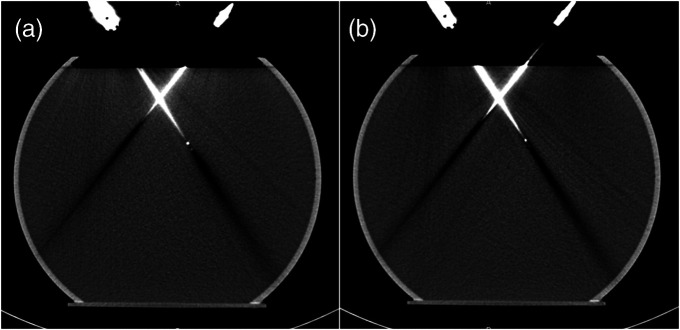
Comparison of probes placed coplanar with the imaging plane: (a) real probes and (b) artificially inserted probes (W/L=400/40  HU). Notably, to reveal two probes and their associated artifacts in a single image, the reconstructed CT images (slice thickness: 1.0 mm) were reformatted to 5.0 mm.
